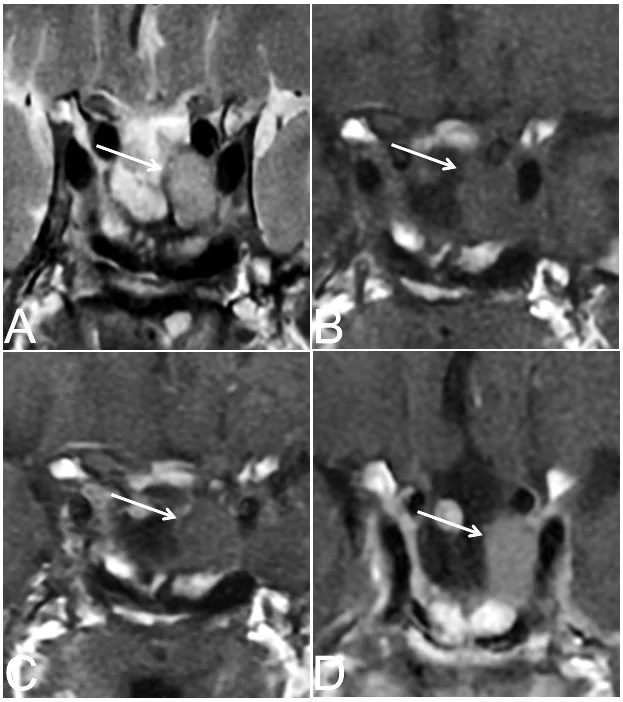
Recurrent Disease. 4 months post transsphenoidal surgery, camewith rising serum IGF-1 (110 ng/mL,previously20 ng/mL). MRI demonstrates a well-defined lesion (arrow) along the left margin of the sella turcica. The lesion appears mildly hyperintense on coronal T2WI (A) and hypointense on coronal T1WI (B). On dynamic assessment, no contrast uptakeis appreciated on the early phase (C), whilediffuse mild homogeneous enhancement demonstarted on the delay phase (D), consistent with recurrent disease.
